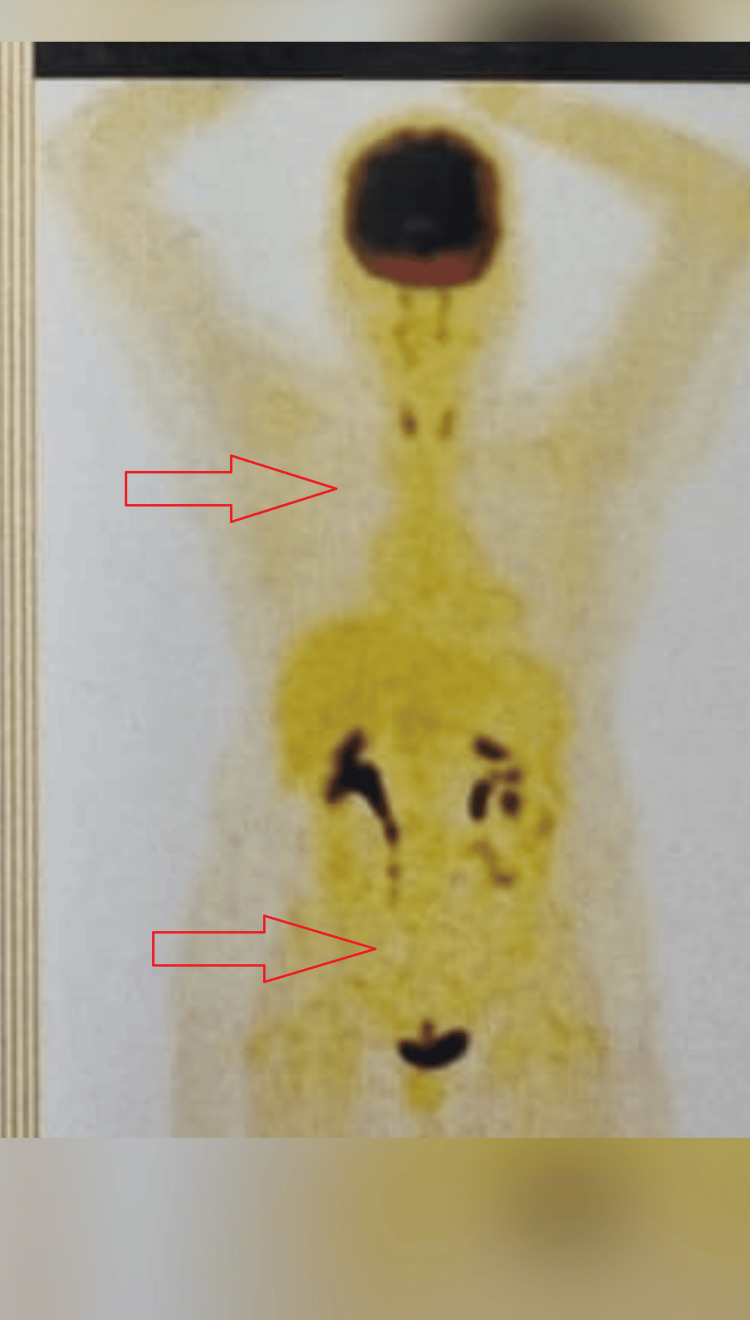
Annual follow-up positron emission tomography-computed tomography scan showing complete metabolic response.
